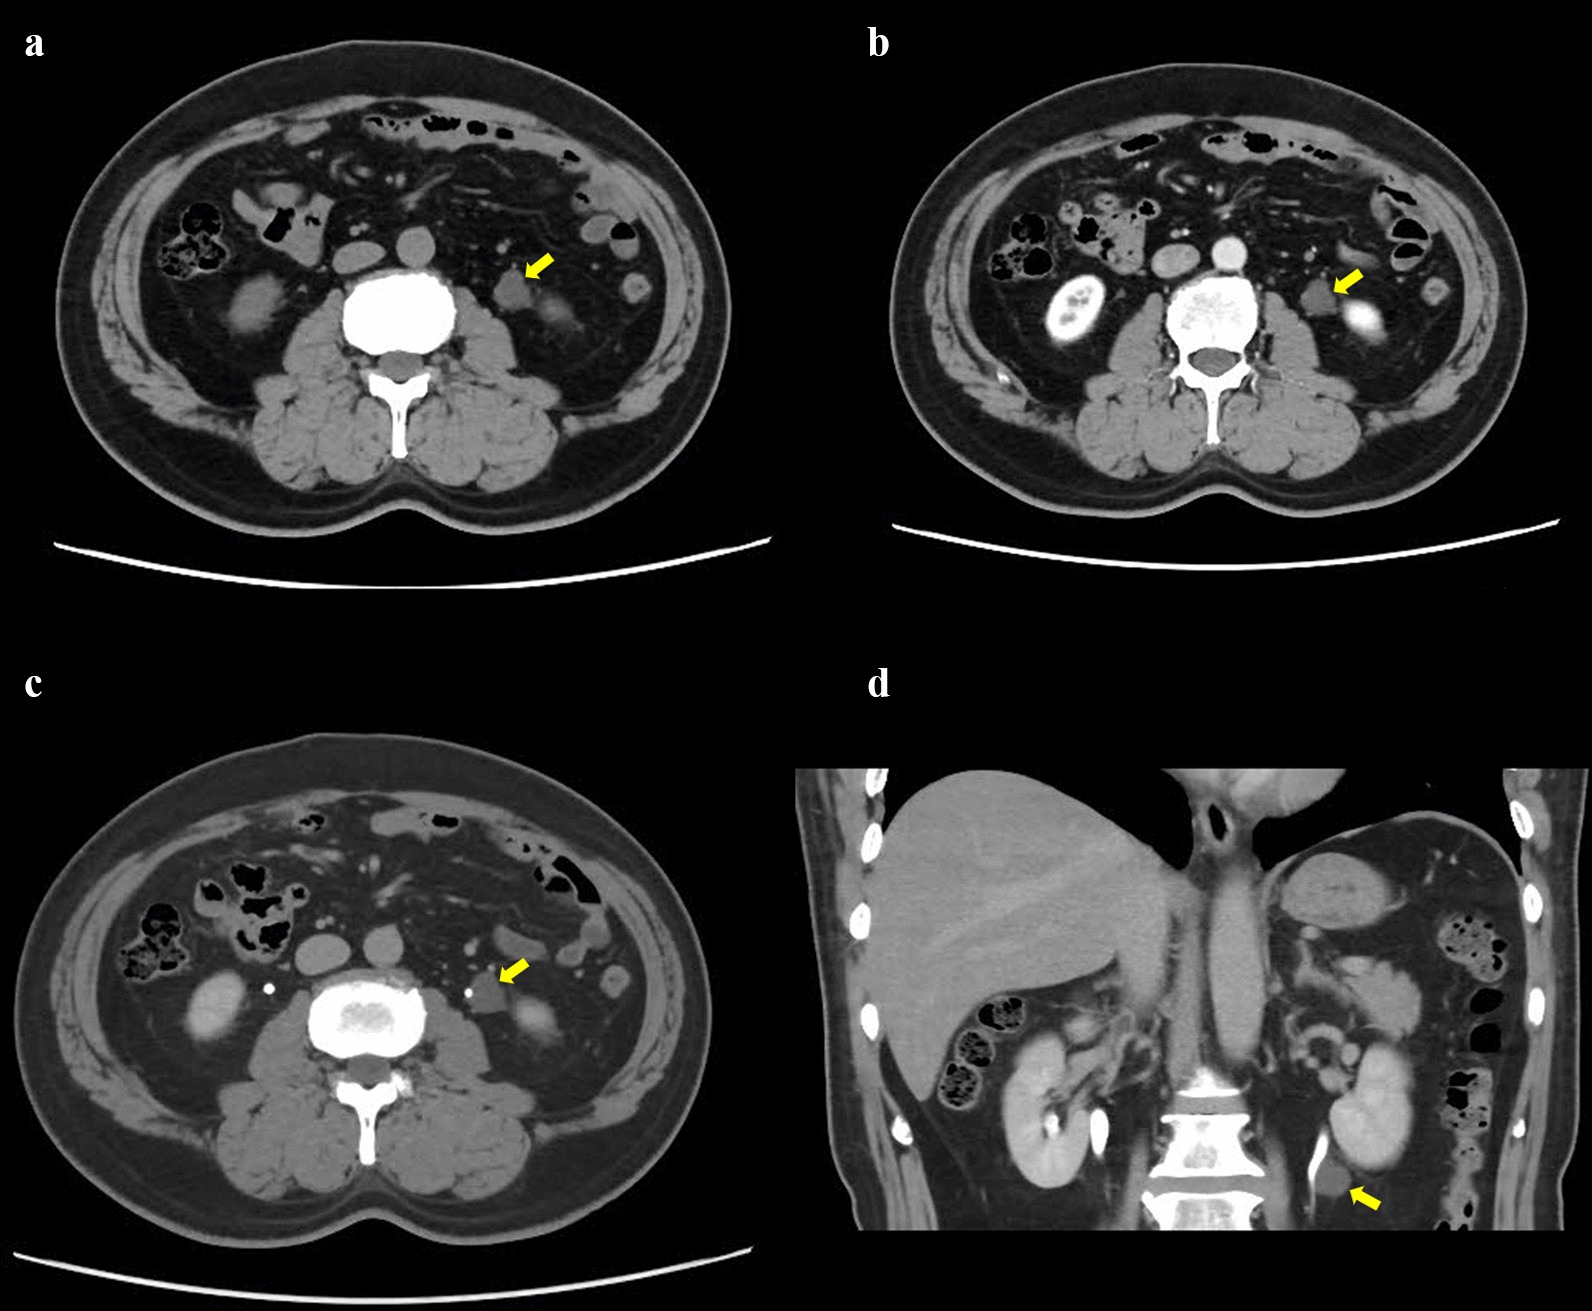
Axial (a–c) and coronal (d) contrast-enhanced CT of the abdomen. CT imaging showed the mass abutting the left ureter was 15 mm in diameter without causing obstruction (a, b), which could been obviously seen in the excretory phase (c). The mass was located between the left ureter and the lower pole of the left kidney (d)
